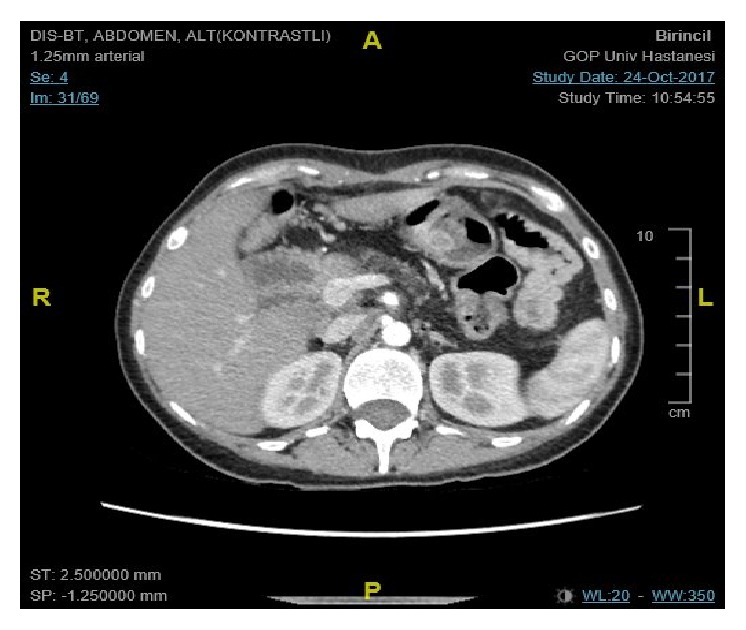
Contrast-enhanced computed tomography 7 months after Whipple procedure. No residual tumor can be seen.
